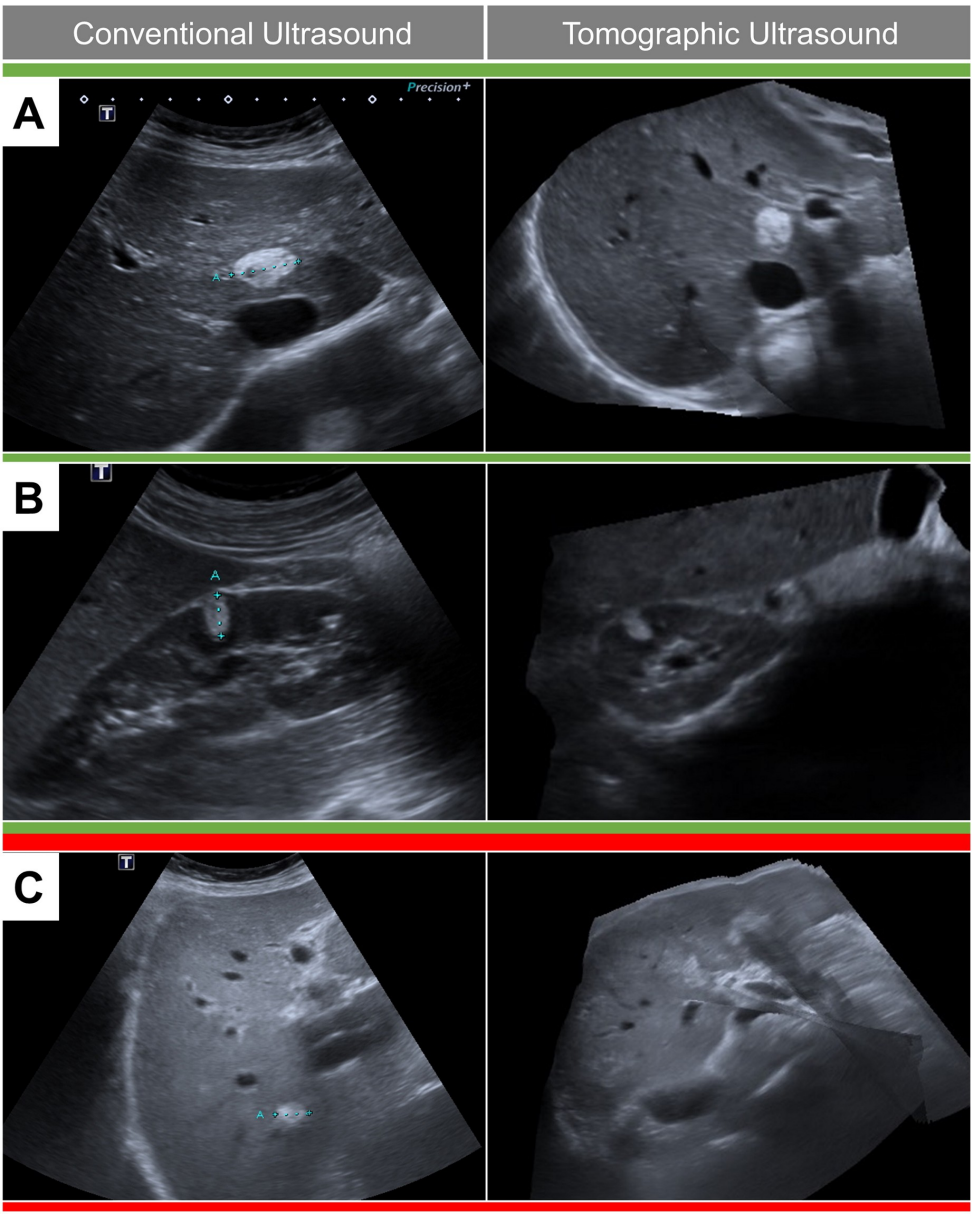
Impact of image quality and artifacts on tomographic ultrasound. Liver and kidney lesion are shown in conventional (left) and tomographic (right) ultrasound. Panels A and B represent examples with good image quality of the tomographic reconstruction. Panel C shows artifacts in the reconstructed image (right) which impairs the detection of the small hyperechoic lesion.
